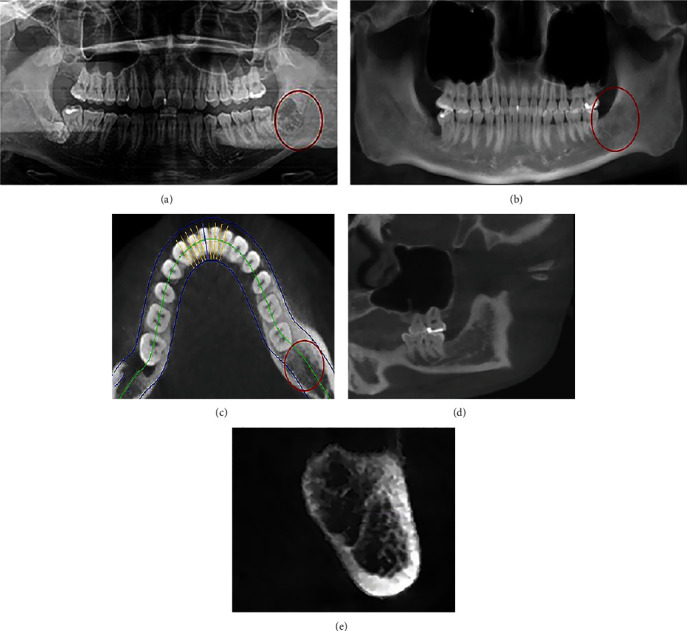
(a) Immediate postoperative panoramic examination showed the empty tooth socket; (b) after six months, CBCT examination showed good bone formation, and the alveolar bone healed well. (c) Transverse section shows increased bone density in empty alveolar bone; (d, e) Coronal and sagittal plane shows increased height of the alveolar bone.
